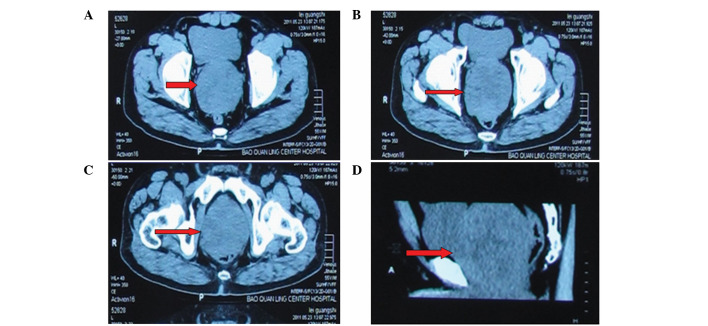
Computed tomography images at four different levels demonstrated a large prostate; size, 10×10.5×9.5 cm. The arrows indicate the tumor.
